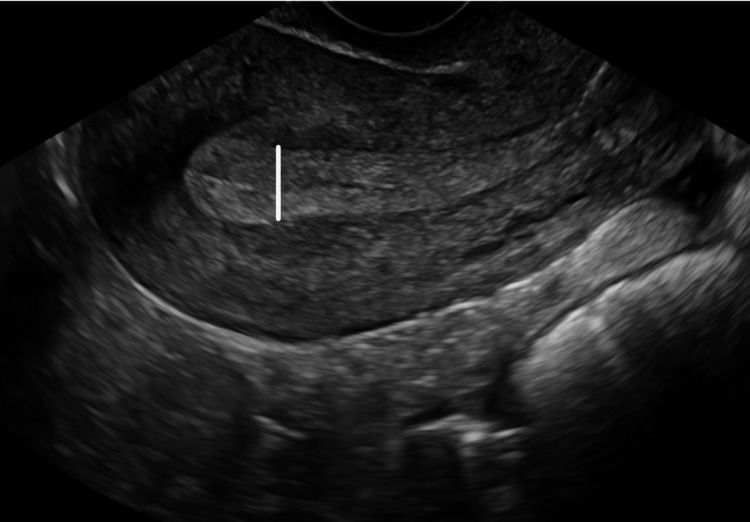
Ultrasonography showing thickened endometrium (white line)
